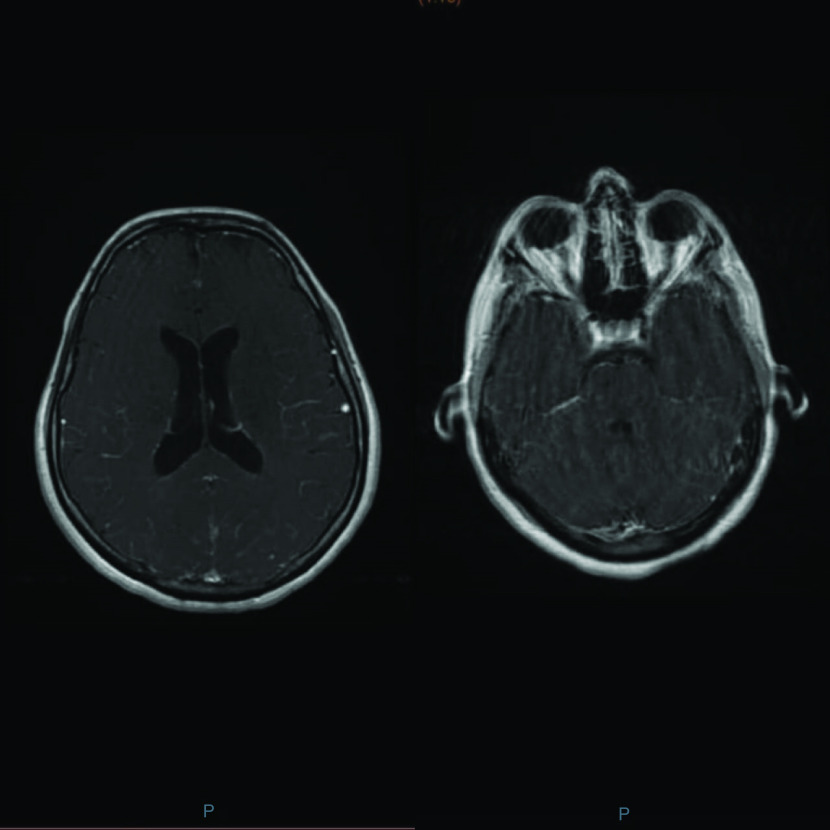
An MRI of the brain showing a diffuse leptomeningeal enhancement with hyperemia, compatible with the signs of meningitis.
